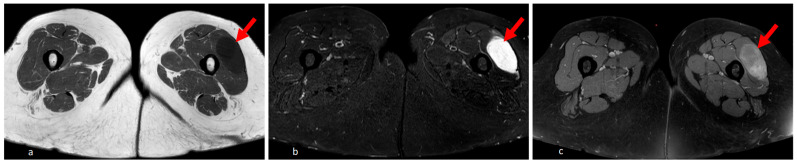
Axial MRI images of an intramuscular myxoma in the vastus lateralis muscle. The lesion appears well-defined and hypointense on the T1-weighted image (a), hyperintense on the T2-weighted sequence (b), and shows inhomogeneous enhancement after contrast administration (c). The arrow highlights the intramuscular myxoma.
